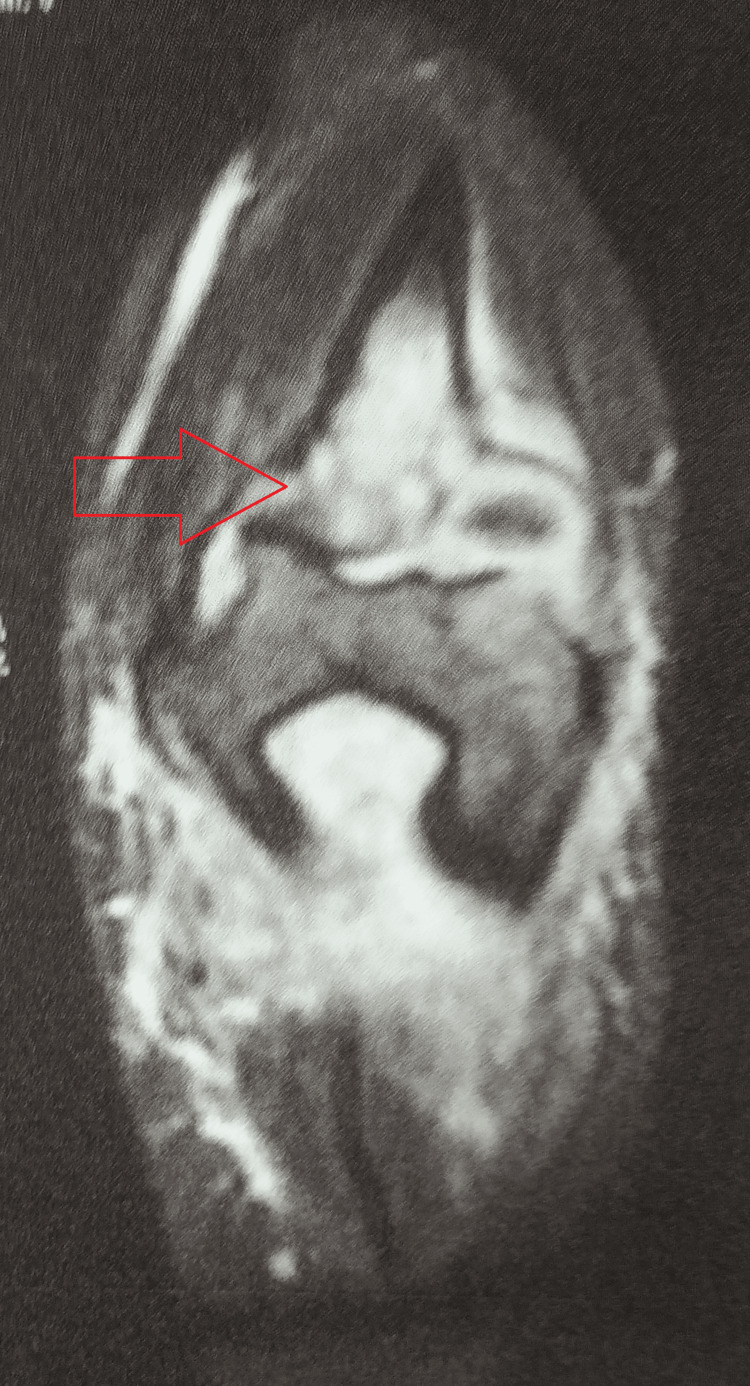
MRI of the right elbow joint suggestive of subarticular erosive changes in the bones forming the elbow joint MRI: magnetic resonance imaging
